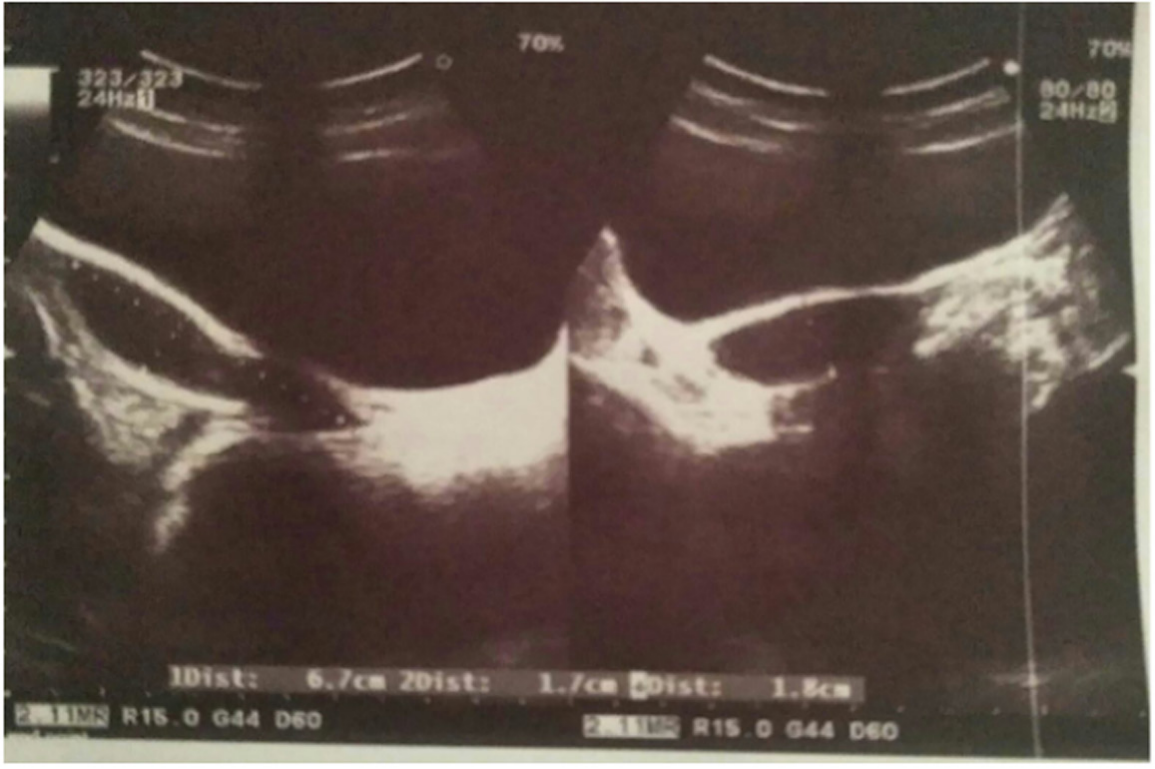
Sagittal and axial transabdominal ultrasound shows a small uterus with thinning of the myometrium
